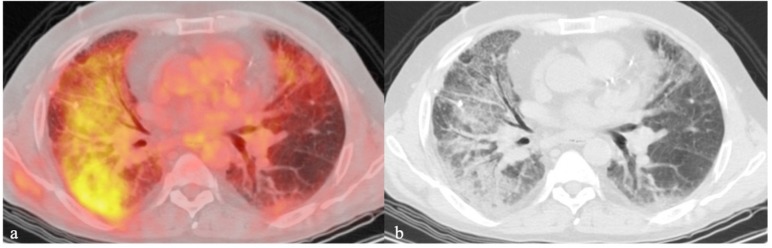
A 60-year-old male with end-stage interstitial lung disease examined by ^18^F-FDG-PET/CT, which demonstrates the high metabolism (a) of the affected pulmonary areas (b).
